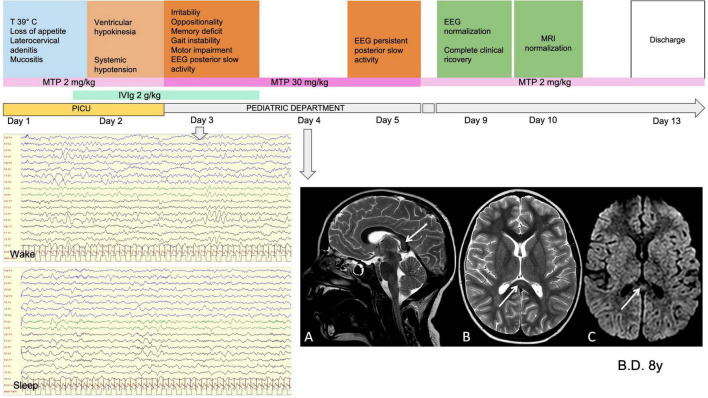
Clinical, MRI and EEG findings in representative patients with focal EEG alterations. Male, 8 years old. MISC presentation characterized by fever (T 39^°^C), systemic symptoms, ventricular hypokinesia and neurological symptoms (irritability, oppositionality, memory deficit gait instability, motor impairment). WBC 16460 (10^A^3/mmc) (N 14579, L 1030); PLTs 152 (10^A^3/mmc); Hb 10.1 (g/dL); CRP 189.6 (mg/l); Ferritin B29 (mcg/l); D-dimer 1114 (mcg/l); NT-ProBNP 15979 (ng/l); TnT 28 (ng/l). On day 1 he was given Mg (2 g/kg for 2 days) and on day 2 was started on high-dose MTP (30 mg/kg for 3 days). MRI performed on day 4 (A). Sagittal, (B) Axial T2-weighted images show slight focal median hyperintense lesion in the splenium of corpus callosum (arrows). (C) Axial diffusion-weighted image shows reduction of proton diffusivity at the site of the lesion (arrow). EEG performed on day 3 (D). High-amplitude delta slow activity prevalent in the posterior regions (E). Slow activity during sleep with preserved spindles. Clinical and EEG normalization was observed on day 7 and normalization of MRI on day 9. CK, creatine kinase; WBC, white blood cells; N, neutrophils; L, lLymphocytes; PLTs, platelets; Hb, hemoglobin; CRP, C-reactive protein; NT-ProBNP, amino-terminal fragment of the brain natriuretic peptide; TnT, troponin T; IVIg, intravenous immunoglobulin; MTP, methylprednisolone; MRI, magnetic resonance imaging; CC, corpus callosum; EEG, electroencephalography; PICU, pediatric intensive care unit admission.
